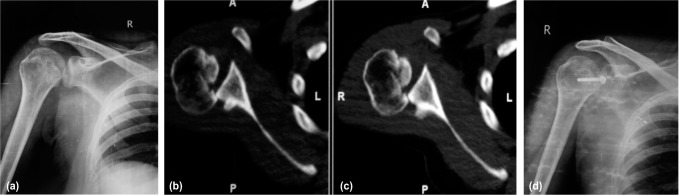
(a) Radiographs of a 21-year old male showing posterior dislocation of the shoulder. It was neglected and patient presented to us at 5 months post injury. (b) CT scans showing engaging reverse Hill-Sachs lesion. (c) Radiographs of the same patient post surgery at 2 years follow up.
